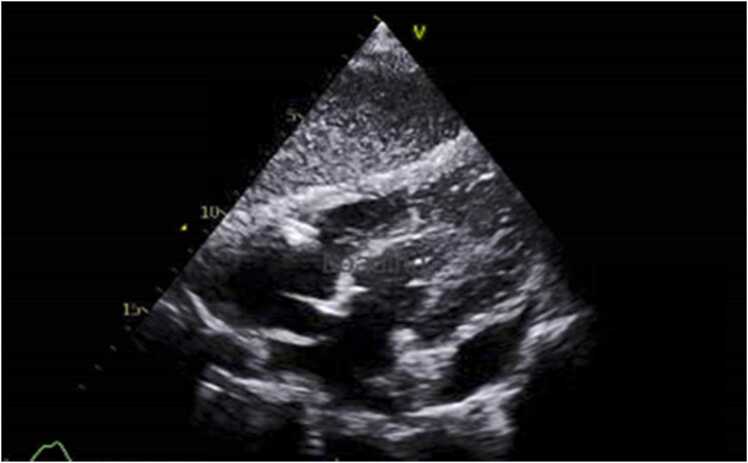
Echocardiogram showing loculated pericardial effusion measuring around 2 cm with no signs of tamponade.
